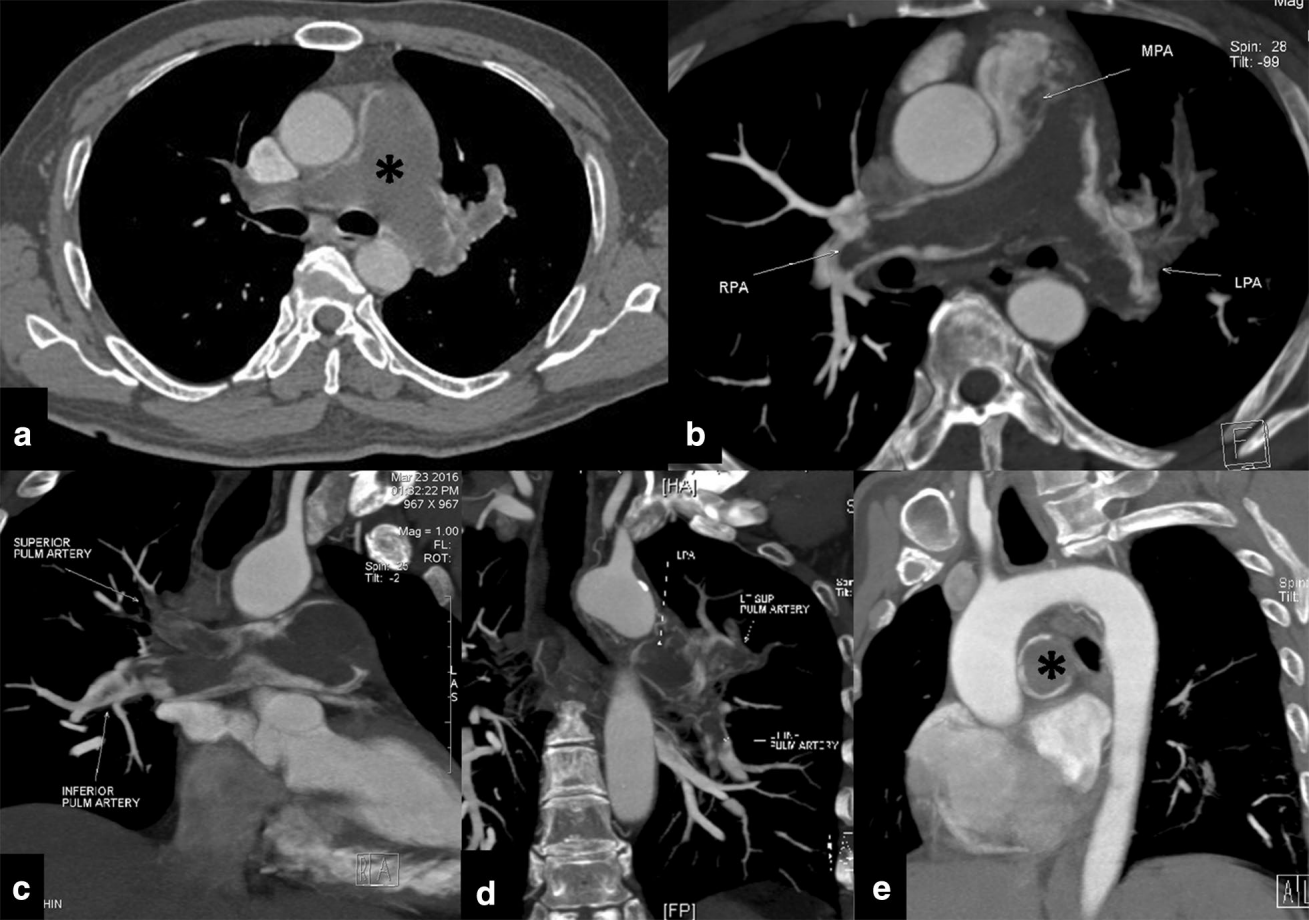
Contrast enhanced CT imaging of the thorax (a, b axial; c, d oblique coronal; e sagittal reformatted images) shows a hypodense filling defect (*asterisk*) in the main and bilateral pulmonary arteries which does not exhibit post contrast enhancement (*MPA* main pulmonary artery, *RPA* right pulmonary artery, *LPA* left pulmonary artery). No pulmonary parenchymal abnormality was noted
